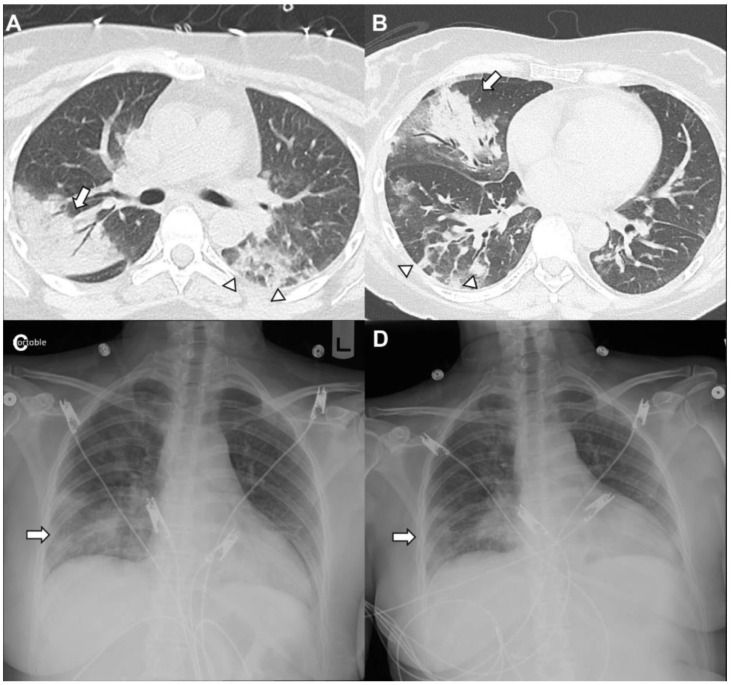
Cryptogenic organizing pneumonia. Lymphoma treated with obinutuzumab and lenalidomide presenting cryptogenic organizing pneumonia. Computed tomography of the chest (A) revealed consolidative opacities in the right upper lobe (arrow) and ground-glass (arrowhead) peripheral infiltrates on the left. She returned 2 weeks later with low-grade fever and cough; repeat imaging (B) showed bilateral multilobar ground-glass (arrowheads) and consolidative opacities (arrow) in the peribronchovascular and peripheral distribution (right greater than left) with a migratory pattern. Chest radiograph on readmission (C) showed infiltrates (arrow) in the right middle and lower lobes which improved days later (D, arrow) after steroid therapy.
